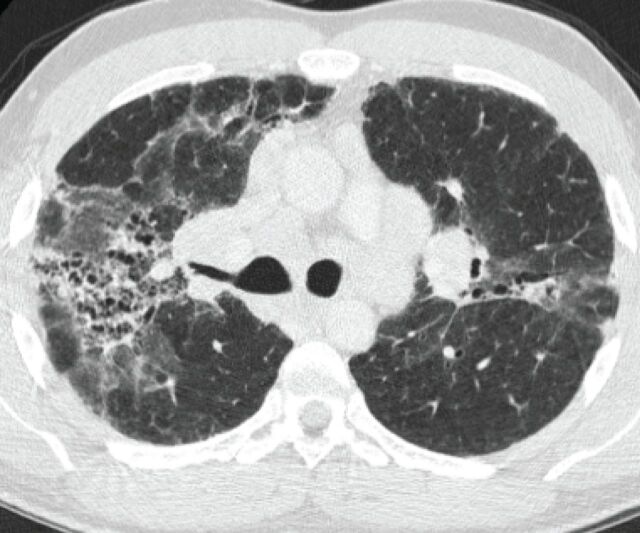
High-resolution computed tomographic section of a patient with active fibrotic sarcoidosis, with typical appearance of peri-hilar fibrocystic changes and accompanying ground-glass changes. Several patterns of fibrosis can be found but accompanying ground-glass changes could signify concomitant presence of cellular infiltrate or fine fibrosis.
